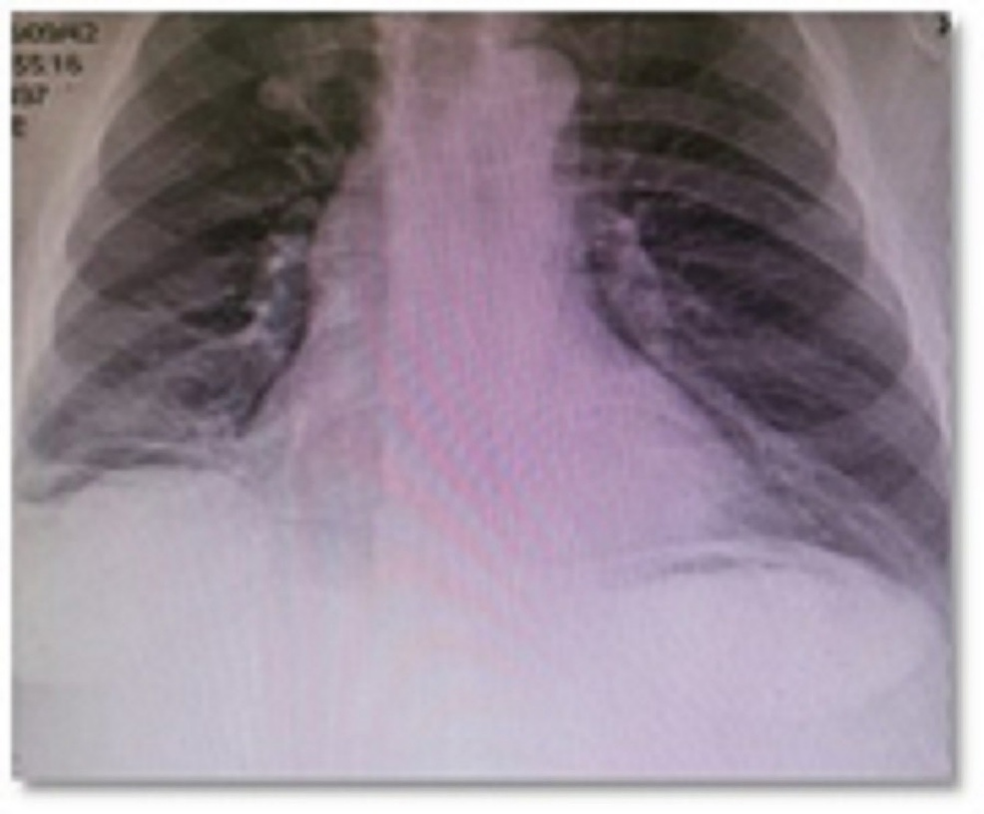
Chest X-ray showing pneumoperitoneum suggesting viscus perforation
